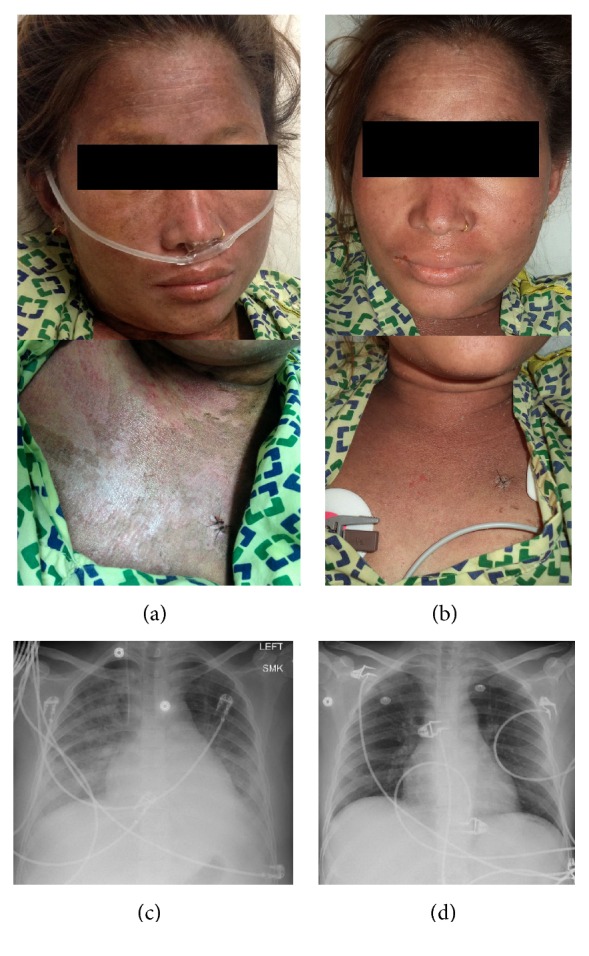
Photograph of the rash and chest X-ray at presentation and discharge. (a) Face and neck upon presentation. (b) Face and neck upon discharge. (c) Chest X-ray at presentation showing bilateral infiltrates. (d) Chest X-ray at discharge showing resolution of infiltrates.
